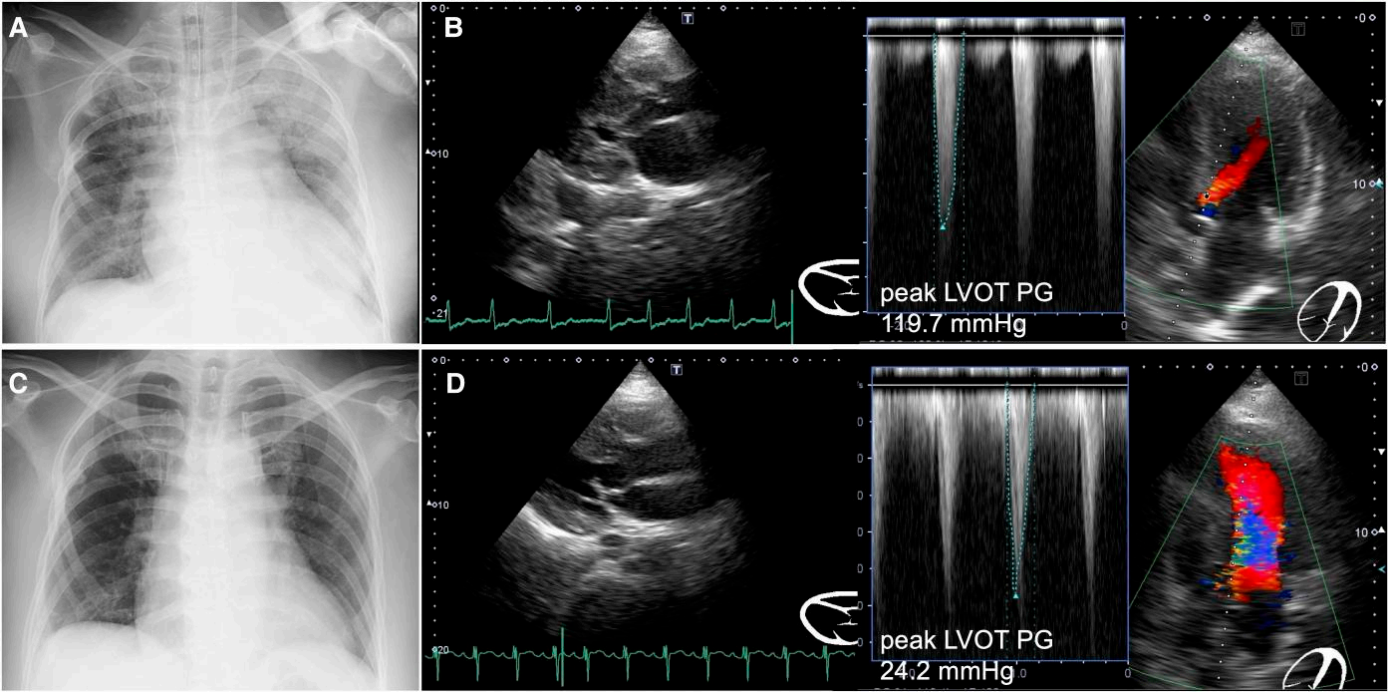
Chest X-ray and transthoracic echocardiography (TTE) before and after percutaneous transluminal septal myocardial ablation (PTSMA). *(A)* Chest radiograph before PTSMA, showing severe cardiomegaly and pulmonary congestion. *(B)* TTE before PTSMA indicates stenosis of the left ventricular outflow tract (LVOT) in the systolic axial view (left panel) and peak LVOT pressure gradient of 119.7 mmHg in the apical four-chamber view (right panel). *(C)* Chest radiograph after PTSMA, showing a decreased cardiothoracic ratio. *(D)* TTE after PTSMA indicates stenosis at the LVOT in the systolic axial view (left panel) and a peak LVOT pressure gradient of 24.2 mmHg in the apical four-chamber view (right panel). LVOT PG, left ventricular outflow tract pressure gradient.
